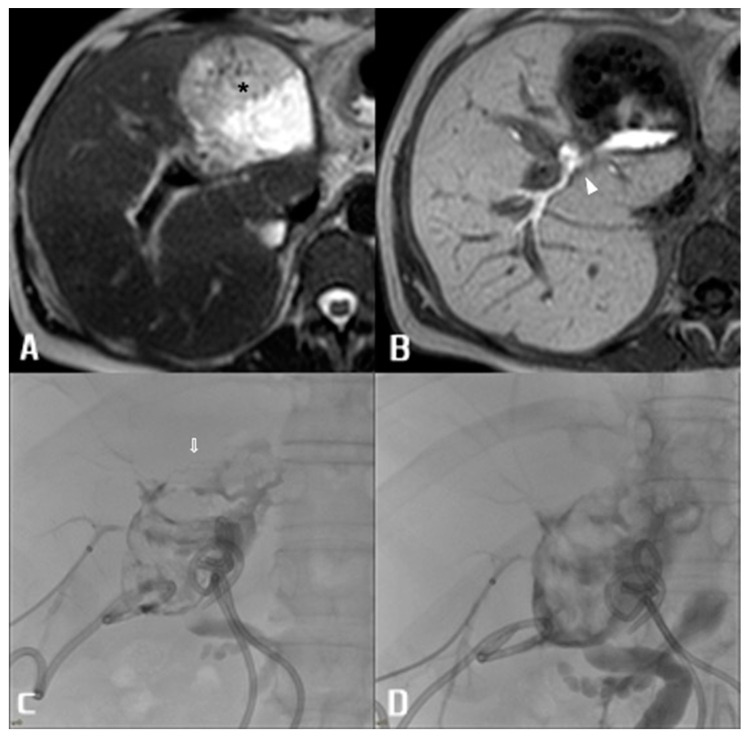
39 y.o. female patient with split right liver transplant. (A) T2-weighted axial image depicts large peri-hilar biloma with inhomogeneous signal (arrow). (B) T1-weighted images obtained after 20 min administration of c.m. show extravasation of c.m. in the biloma at the origin of the right hepatic duct (arrowhead). (C,D) Percutaneous cholangiography performed after puncture of subsegmentary duct of V segment confirmed leakage and biloma opacification with delayed advancement of c.m in the common bile duct of the recipient.
